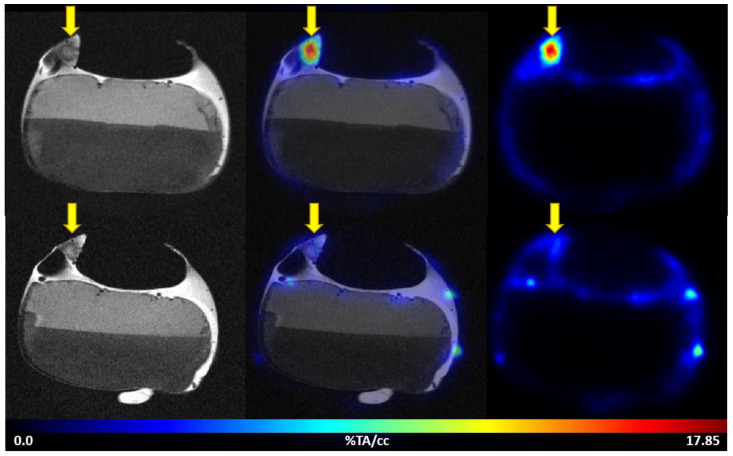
Representative images of [^68^Ga]Ga-Pentixafor PET/MRI in the HT29 CAM-xenograft model. Upper row: baseline scan after i.v. injection of 3.50 MBq [^68^Ga]Ga-Pentixafor (0.47 nmol peptide) on EDD15. Lower row: blocking (315 µg AMD3100/egg) of [^68^Ga]Ga-Pentixafor uptake (7.57 MBq, 0.93 nmol peptide) in the same subject on the following day (EDD16). Left: T2-weighted TurboRARE images, right: [^68^Ga]Ga-Pentixafor PET 60 min p.i., middle: fused PET/MRI (axial planes are shown). HT29 CAM-xenografts are marked with a yellow arrow.
